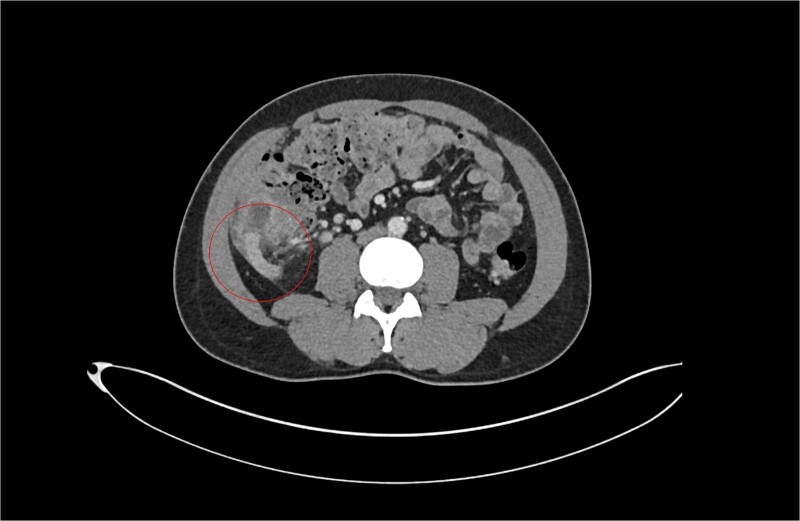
Patient A. Appendiceal stump of Patient A identified within the red circle on CT scan before the second operation.
